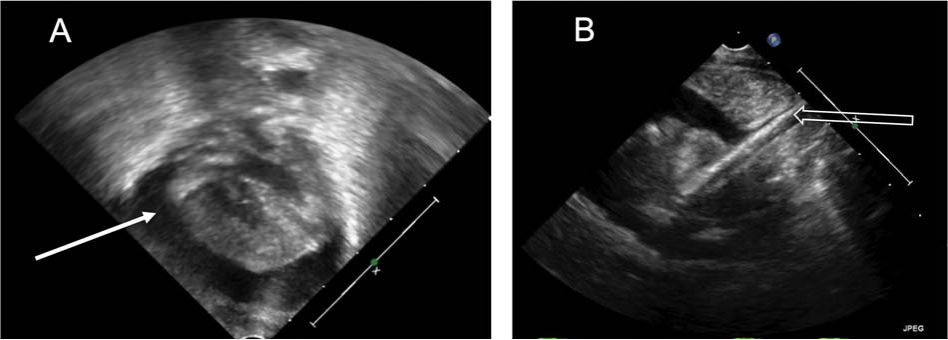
Echocardiogram. (A) Apical view, pericardial effusion (white solid arrow). (B) Subcostal view, ECMO catheter tip seen entering the right atrium (white hollow arrow).
